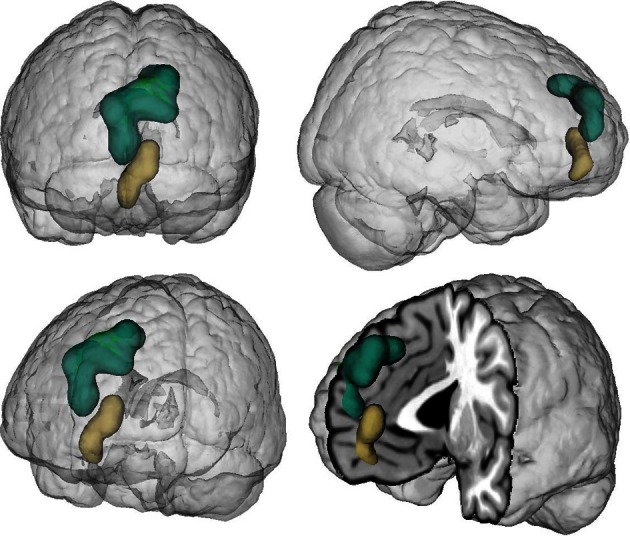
Location of the seed regions. Seeds were drawn from an earlier coordinate-based neuroimaging meta-analysis on perspective-taking, which yielded two clusters of convergent brain activity in the ventral (beige) and dorsal (green) medial prefrontal cortex (Bzdok et al., 2012c). The centers of mass of the vmPFC and dmPFC seed are −4/52/−2 and −6/56/30, respectively. These two seeds represent a functional-structural segregation in the medial prefrontal cortex related to higher social-cognitive processing and provided the basis for the present quantitative analyses. The seeds were rendered into a T1-weighted MNI single subject template using mango (multi-image analysis GUI; http://ric.uthscsa.edu/mango/).
